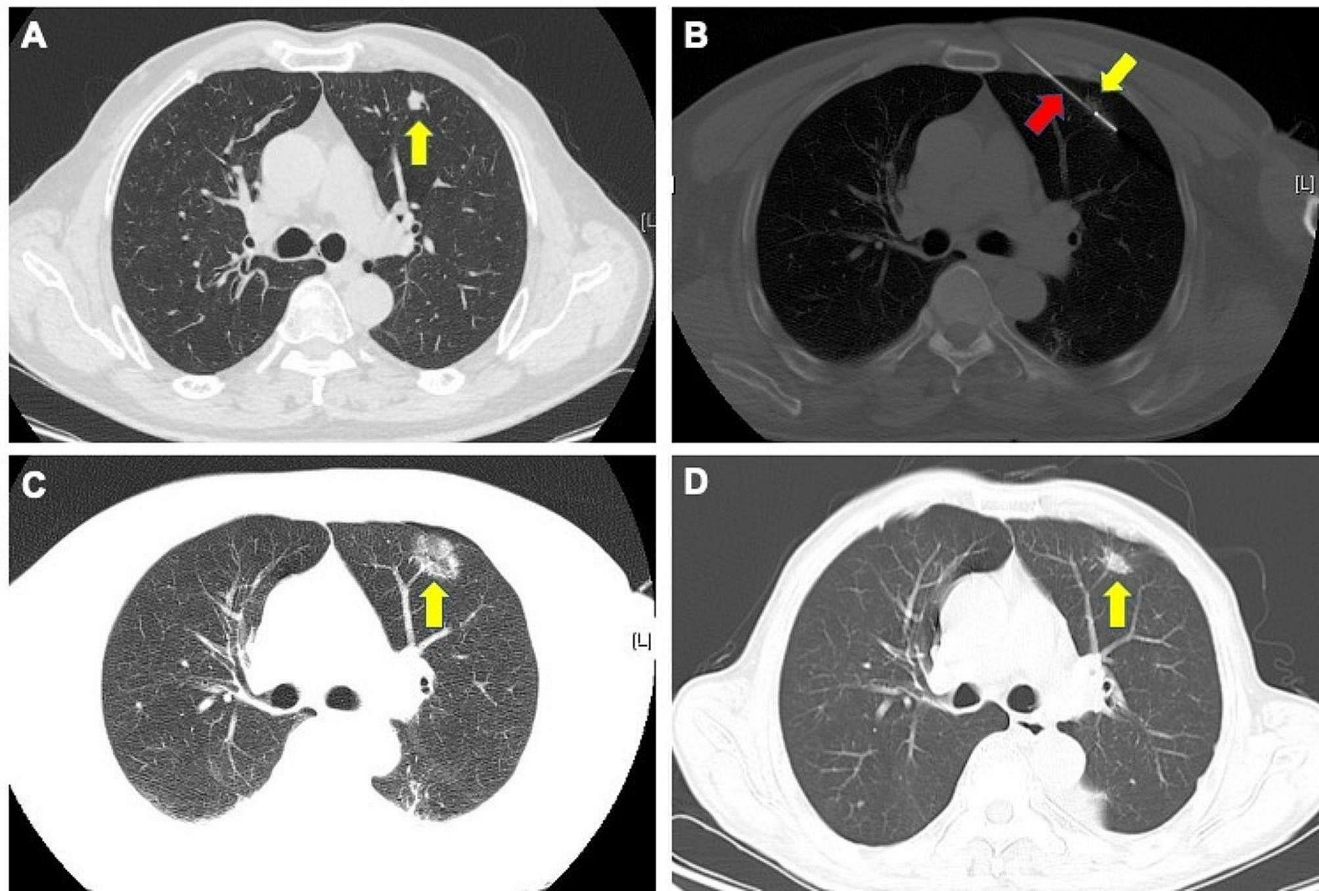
A representative case of MWA. A A metastatic lesion located in the left lung (yellow arrow). B Punctured applicator (red arrow) through the center of metastasis (yellow arrow). C A ground-glass opacity (yellow arrow) indicating complete ablation after the procedure. D Follow-up CT at 12 months
